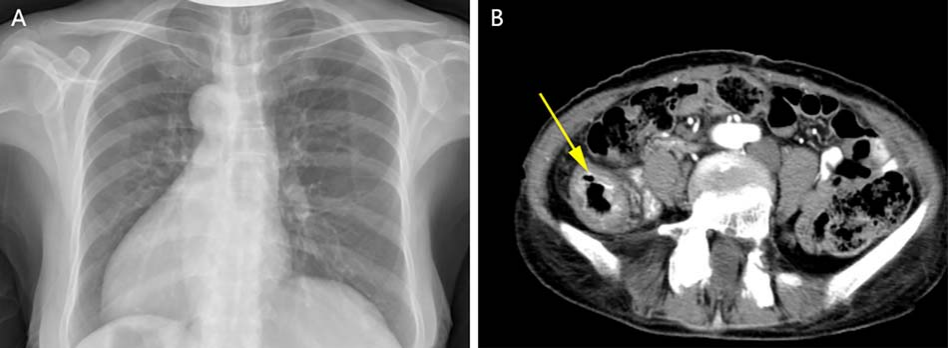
Chest X-ray showed that the heart was on the right side of the chest (A). Enhanced CT of the lower abdomen showed thickening of the descending colon with stenosis and enhancement, which suggested that it might be a tumor of the descending colon (B, yellow arrow).
